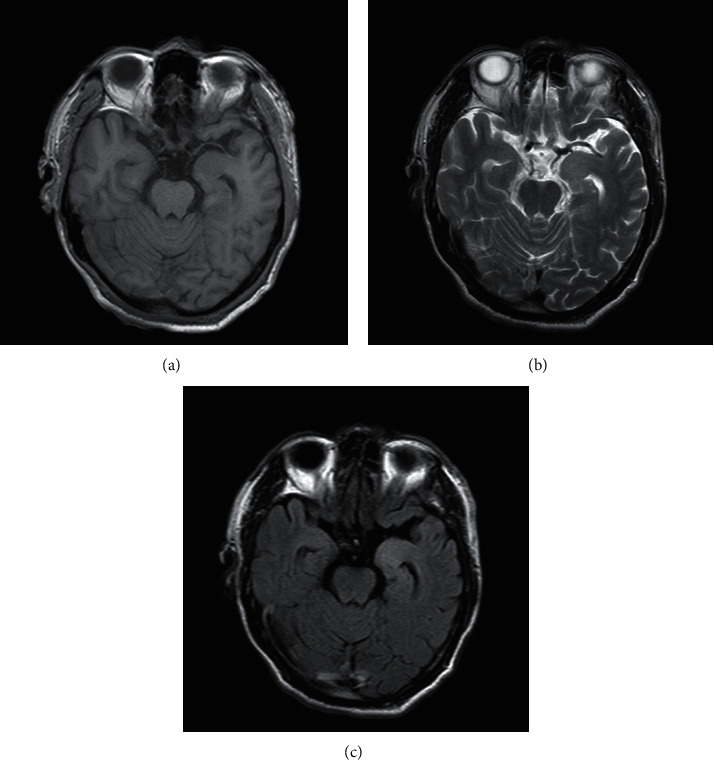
The cranial brain MRI of anti-LG11 antibody-positive encephalitis recurrence in the follow-up: abnormal signal on the left side of the hippocampus, (a) flaky long T1, (b) slightly longer T2 signal, and (c) FLAIR sequence shows a slightly higher signal.
